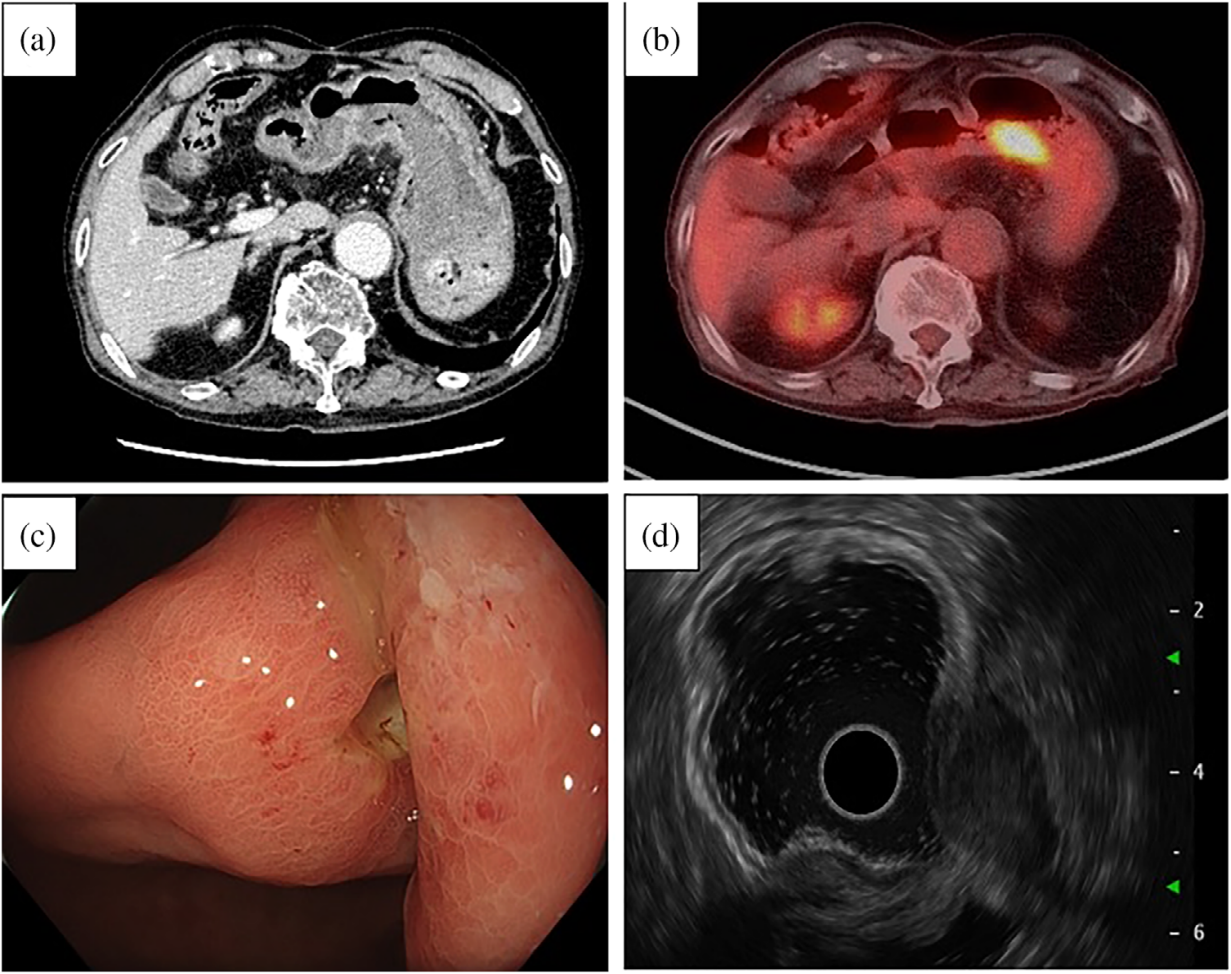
Forty‐five‐month post‐endoscopic ultrasound‐guided fine‐needle aspiration. (a) Contrast‐enhanced computed tomography shows no obvious thickening of the gastric wall or swelling of the surrounding lymph nodes. (b) The upper endoscopy reveals an ulcerative lesion at the same site as the previous biopsy. (c) Positron emission tomography‐computed tomography shows abnormal accumulation in the posterior wall of the gastric angle. (d) EUS shows a 25 mm low‐echoic, irregularly shaped, heterogeneous tumor with clear margins, protruding from the mucosa outside the gastric wall (white arrow).
